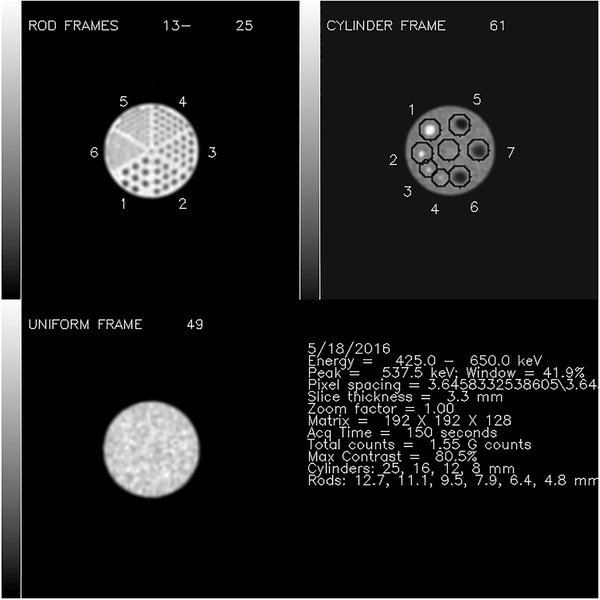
A summary screen reporting the automatically generated QA report for a standard quarterly PET/CT data acquisition
